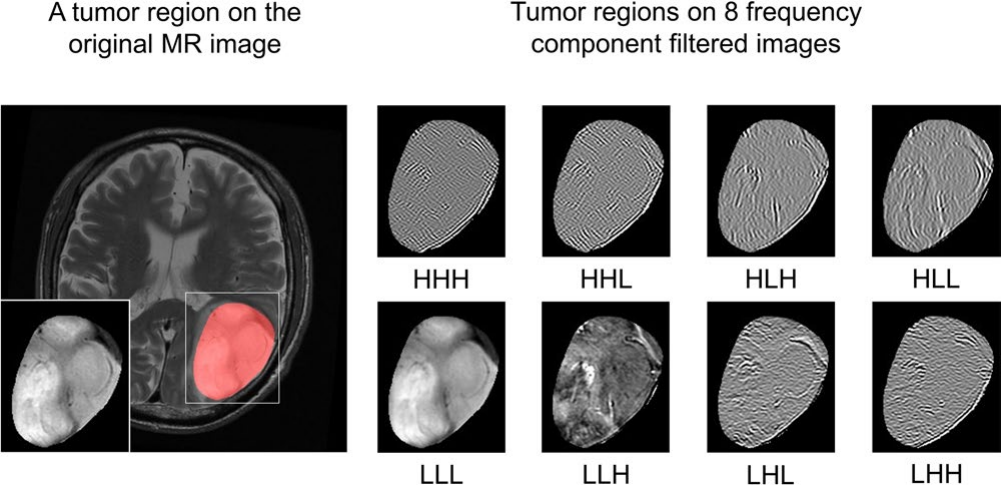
Transverse images of a tumor on original magnetic resonance (MR) image (T2-weighted MR image (T2WI)) and on eight frequency component-filtered images to which a three-dimensional (3D) Coiflet wavelet transform had been applied.
